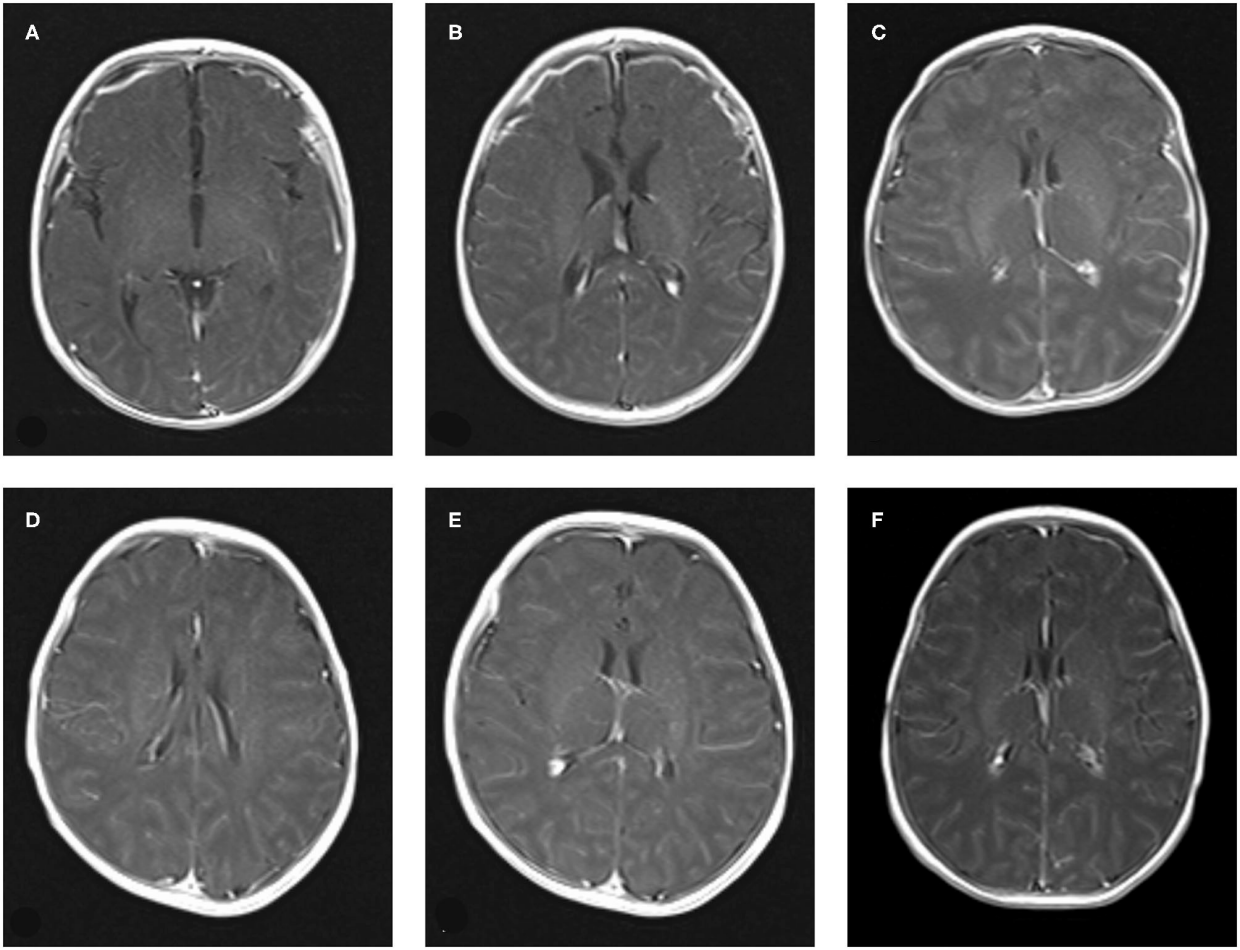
The manifestation of craniocerebral MRI enhanced scan of Neonatal HFMD complicated with encephalitis. (A–C): Bilateral meningeal enhancement. (D–F): The vascular shadow on the surface of the brain increased slightly.
